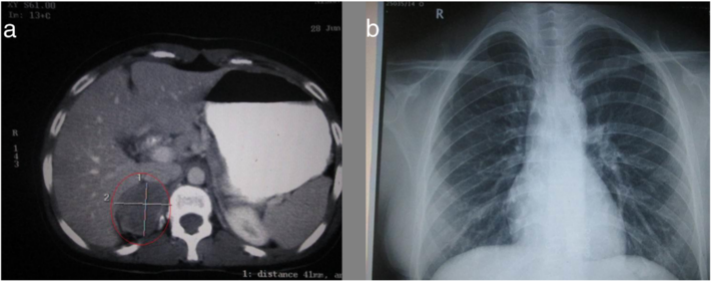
a CT abdomen—note the mass in the right adrenal area. b Plain x-ray chest—within normal limits
